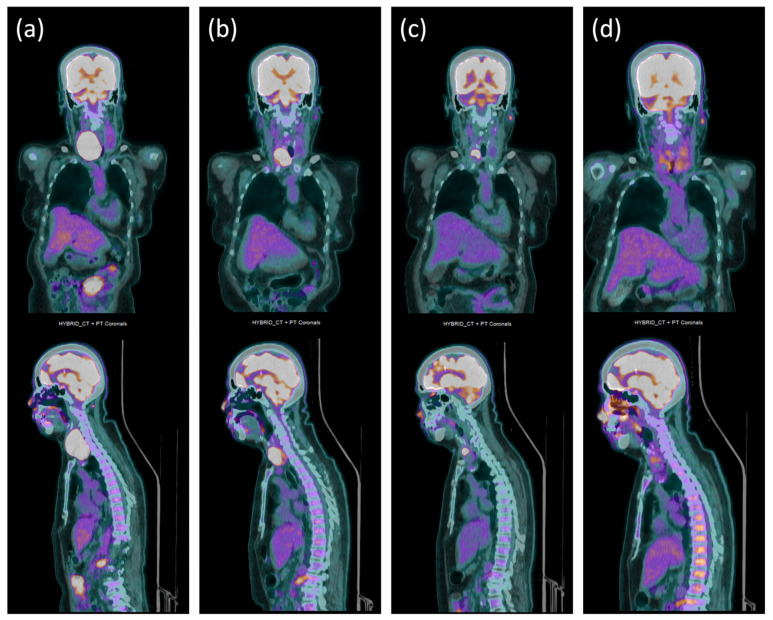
Serial PET/CT scans of Case 4 demonstrating progressive tumor regression following locoregional interventions: (a) baseline image showing a large hypermetabolic right thyroid mass with tracheal compression and deviation; (b) 4 months after TAE; (c) 12 months after TAE and 7 months after the first session of RFA; (d) 20 months after TAE, 15 months after the first session of RFA and 6 months after the second session of RFA.
